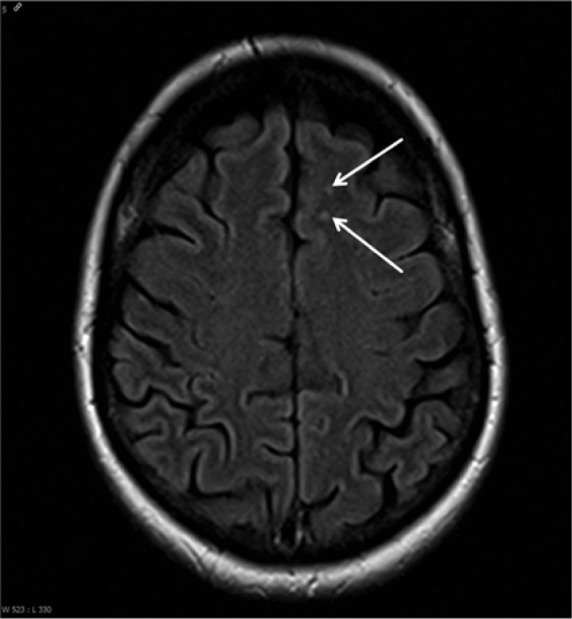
MRI head axial, FLAIR (August 2013): multiple unspecific gliosis spots in the white matter, seen bihemispheric, which can also occur due to neuroborreliosis, exemplarily 2 frontal lesions shown here (arrows).
